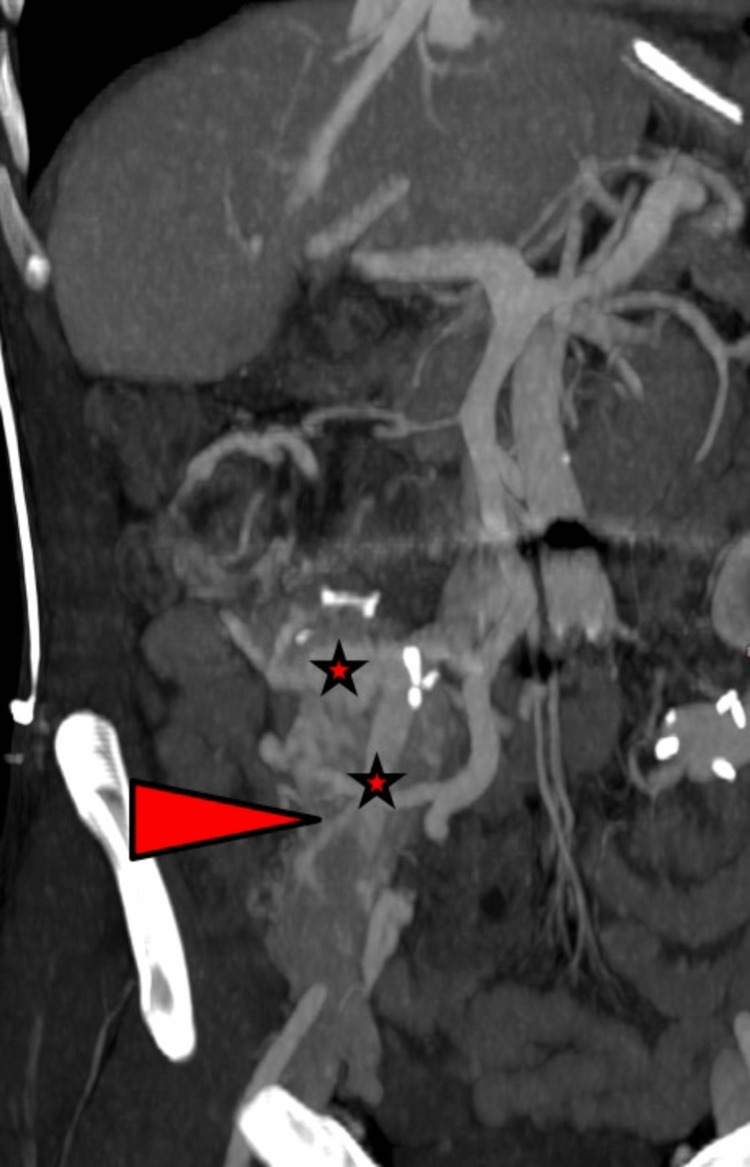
Computed tomography shows extended varicose veins around the pancreas and duodenal graft Arrow-head: pancreatic graft Asterisks: peritransplant varices
